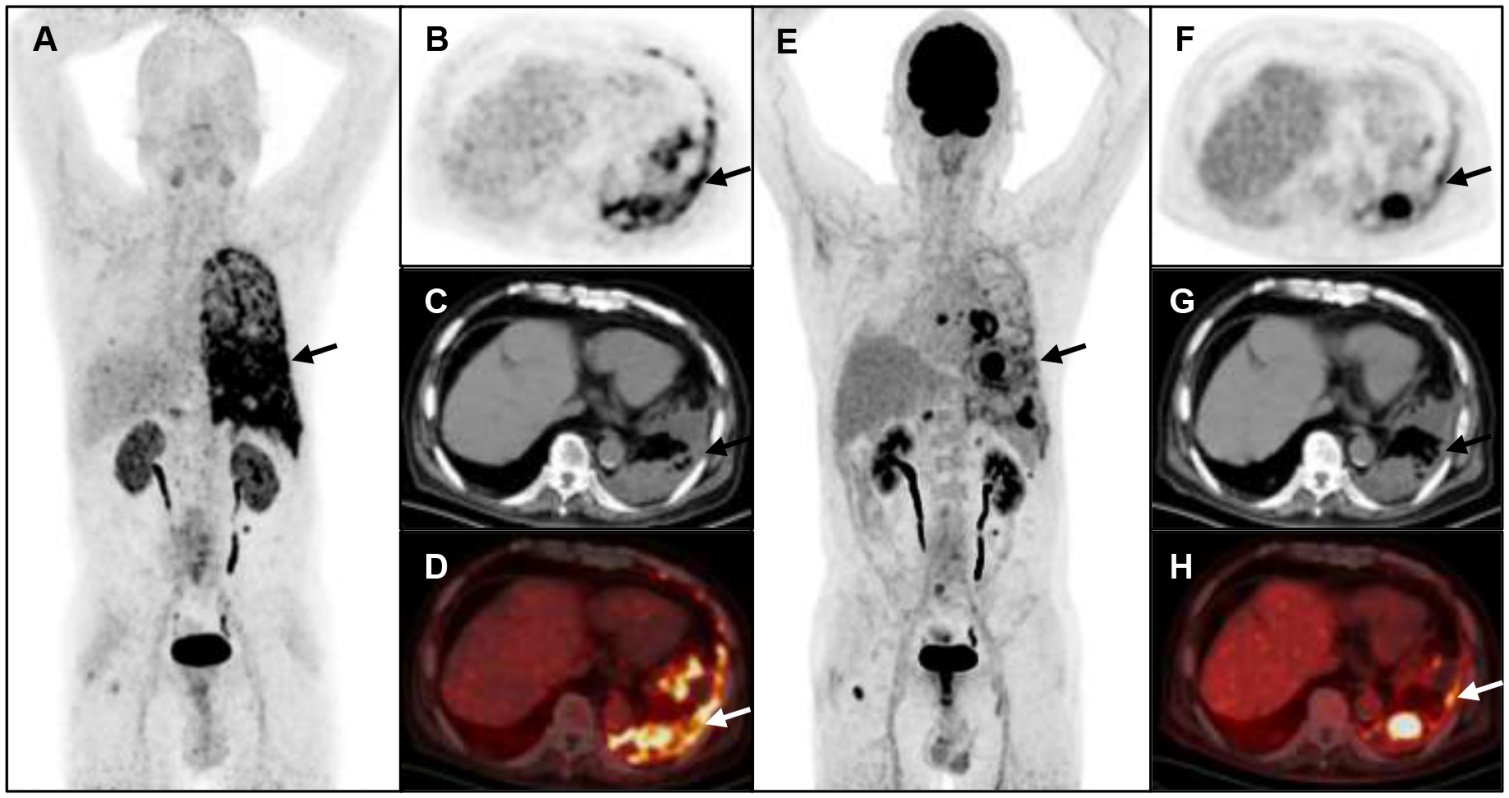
A 75-year-old man with metastatic lung cancer underwent cancer restaging imaging. ^68^Ga-FAP-2286 image shows more metastasis and pleural uptake (A-D), straight arrow, SUVmax 9.83).^18^F-FDG shows lower uptake in pleural (E-H), straight arrow, SUVmax 5.7).
